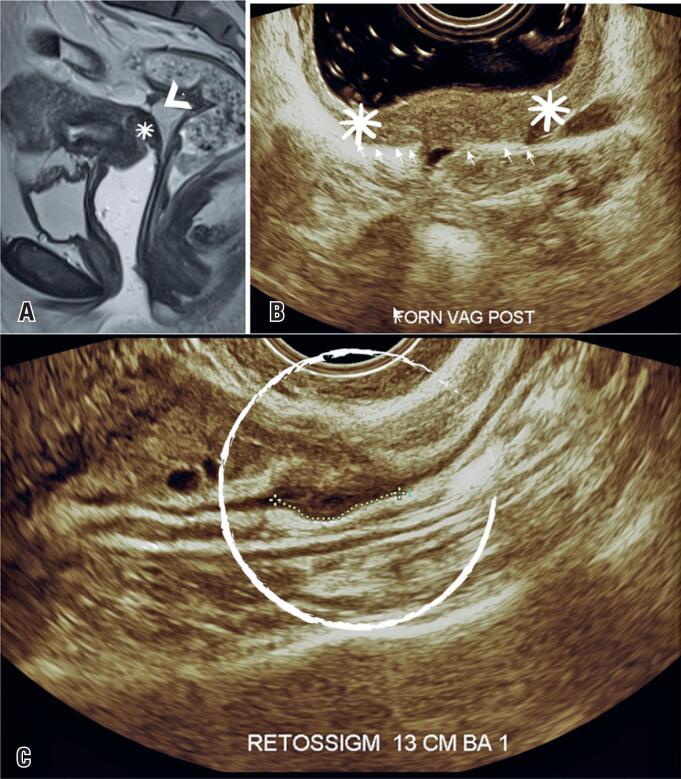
(A) Magnetic resonance imaging of the pelvis in sagittal T2WI (T2-weighted image), with no fat saturation and showing thickening with low signal on the retrocervical region (white head arrow) and vaginal fornix (asterisk), which is related to endometriosis lesions. (B and C) Transvaginal ultrasound with bowel preparation showing invasion of the vaginal mucosa (asterisks) and rectosigmoid deep infiltrating endometriosis (white circle)
